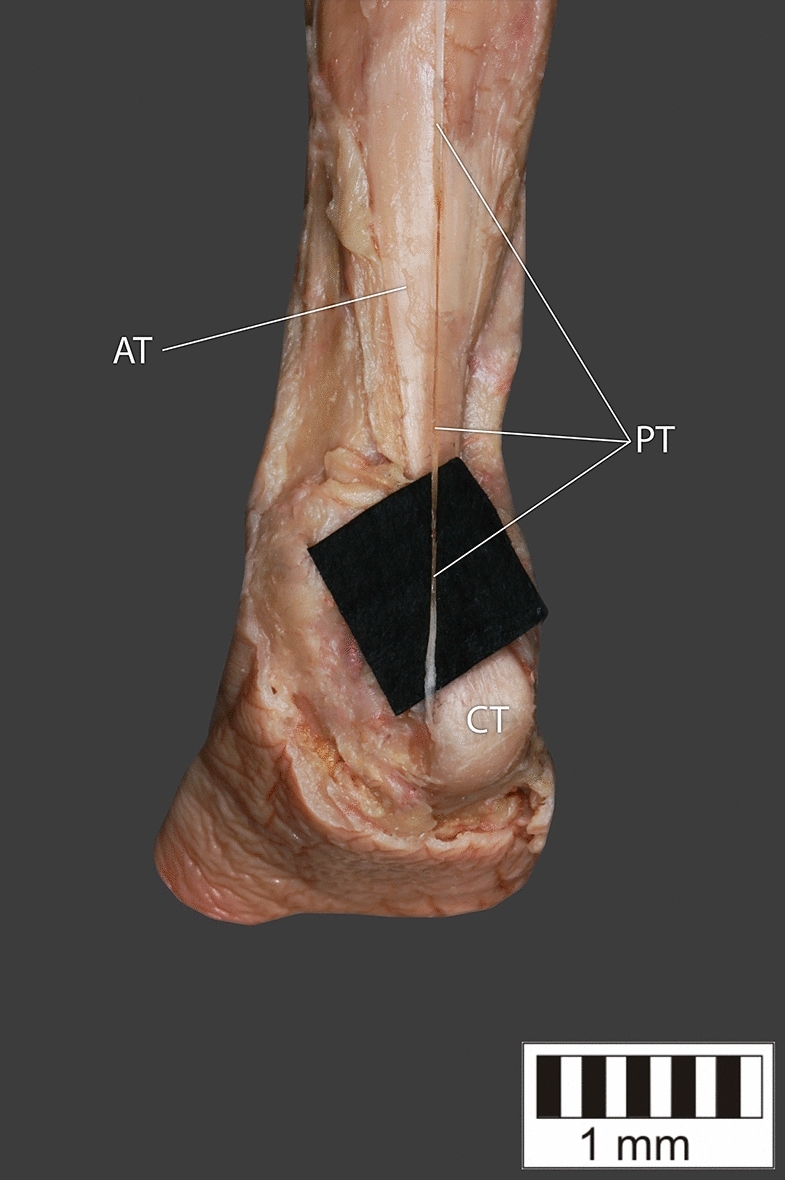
Type II of plantaris tendon. *CT* calcaneal tuberostity, *AT* Achilles tendon, *PT* plantaris tendon.
